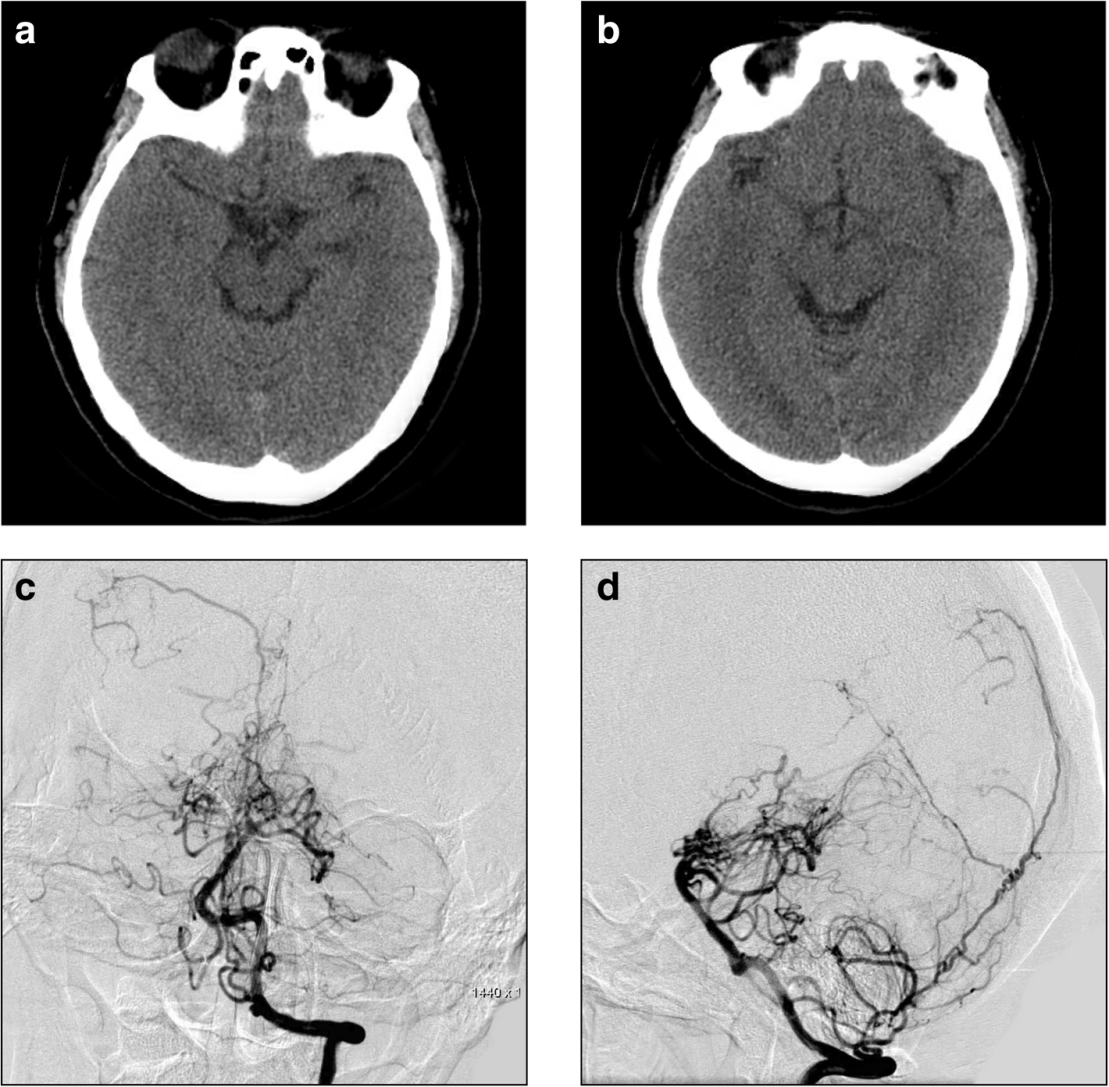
a-b: CT shows complete resolution of the SAH. c-d: Left VA angiogram in AP and lateral views reveals that the aneurysm have disappeared, the distal part of the PCAs become finer and smaller, and the transdural compensation of the posterior meningeal artery could be seen. Abbreviations: AP: anteroposterior; CT: computed tomography, SAH: subarachnoid hemorrhage, PCA: posterior cerebral artery, VA: vertebral artery
